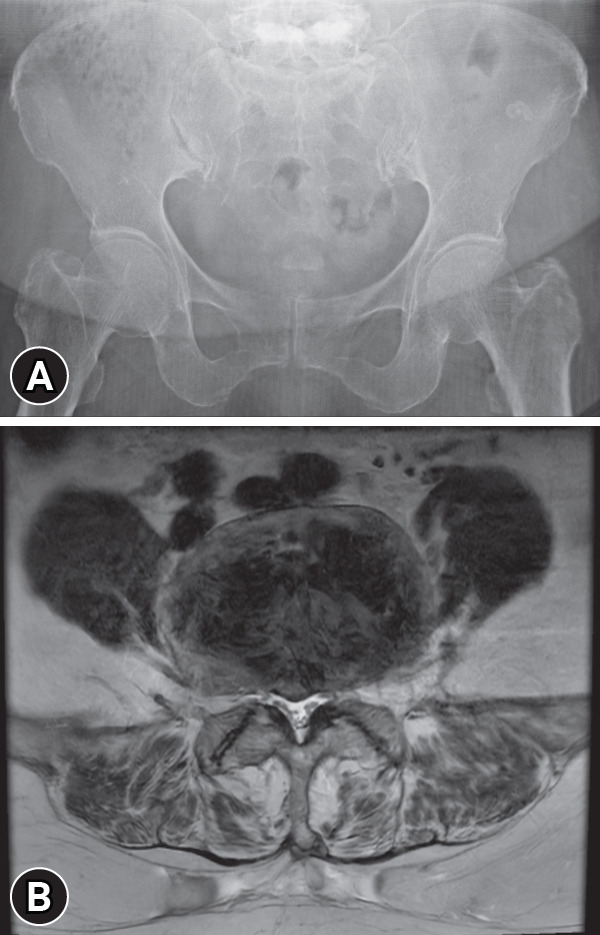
(A) Pelvic radiograph showing degenerative changes in the bilateral sacroiliac joints. (B) Axial T2-weighted lumbar magnetic resonance imaging demonstrating central canal stenosis at L4 to L5.
